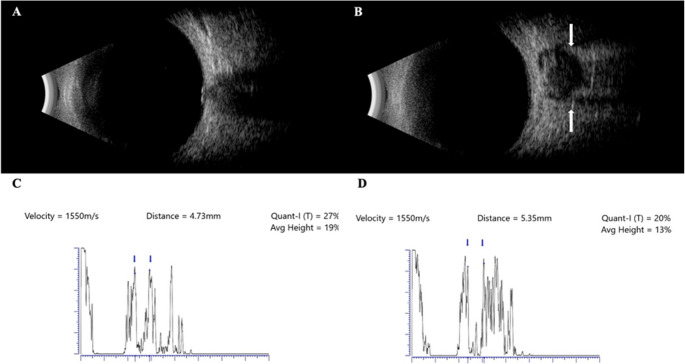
Standardized orbital ultrasound (SOUS) in a patient with spontaneous CSF leak. B-scan showing normal optic nerve preoperatively (A); A-scan showing normal optic nerve sheath diameter (ONSD) preoperatively (C), (B) “crescent sign” due to the subarachnoid liquid (arrow) between the optic nerve parenchyma and perineural sheath, indicative of intracranial hypertension postoperatively and abnormal ONSD after surgical repair of the fistula (D)
